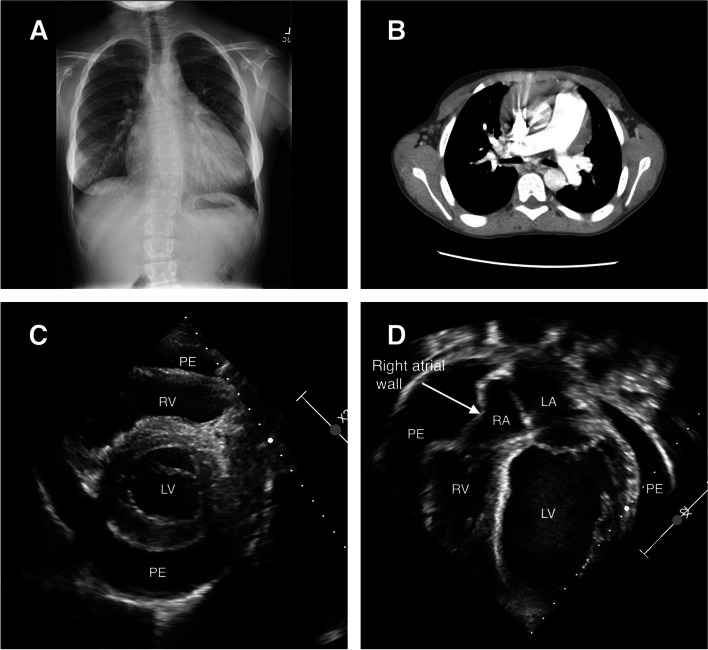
A Chest xray revealing an enlarged cardiomediastinal silhouette, levoscoliosis of the thoracic spine and dextroscoliosis of the lumber spine. B CT pulmonary angiogram showing a dilated pulmonary trunk measuring at least 3.5 c.m with prominent peripheral pulmonary arteries. C Transthoracic echocardiogram in the parasternal short axis view revealing pericardial effusion. D Transthoracic echocardiogram in the apical view depicting pericardial effusion and right atrial wall compression. LA: Left Atrium, LV: Left Ventricle, PE: Pericardial Effusion, RA: Right Atrium, RV: Right Ventricle
